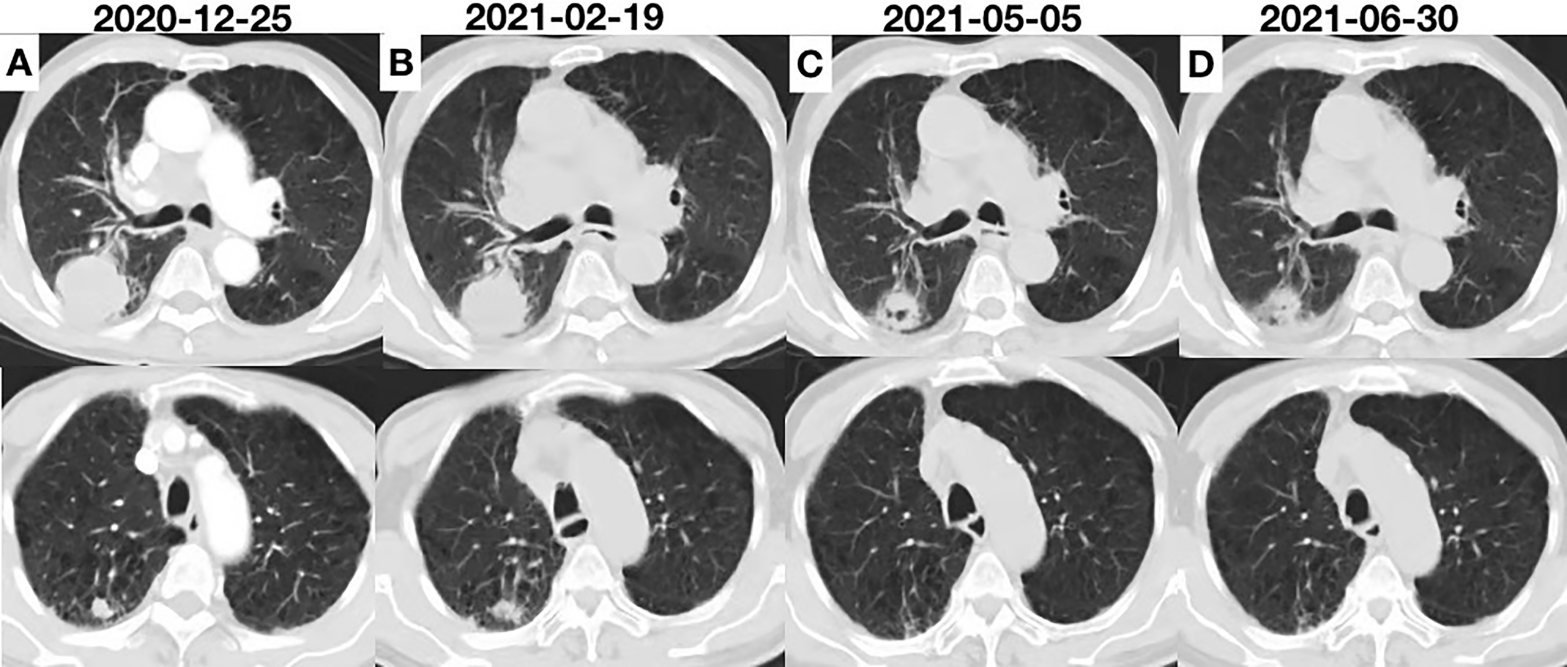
(A) Chest computed tomography (CT) scan showing the initial tumor. (B) Two cycles after anlotinib initiation; the efficacy was evaluated as SD. (C) CT scan showing remarkable shrinkage of the tumor; the efficacy was evaluated as PR. (D) CT scan showing sustained shrinkage of the tumor and a small amount of additional effusion; the efficacy was evaluated as PR.
